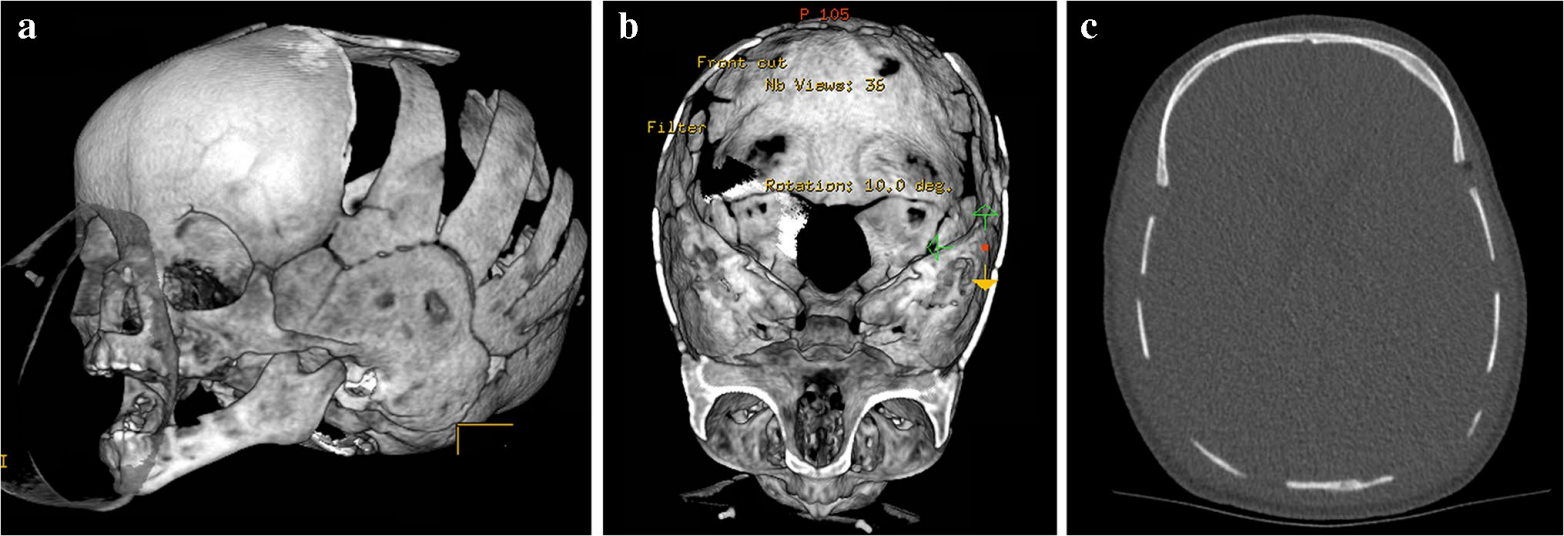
Postoperative non-contrast-enhanced computed tomography (CT) images of a 4-month-old boy with sagittal craniosynostosis.  Three-dimensional surface-rendered (a) intracranial surface-rendered (b) and axial (c) CT images clearly show the cranial sutures. Even though a low-dose CT protocol was used, the conventional multi-slice CT shows the ventricles due to sufficient soft tissue contrast of the imaging technique
